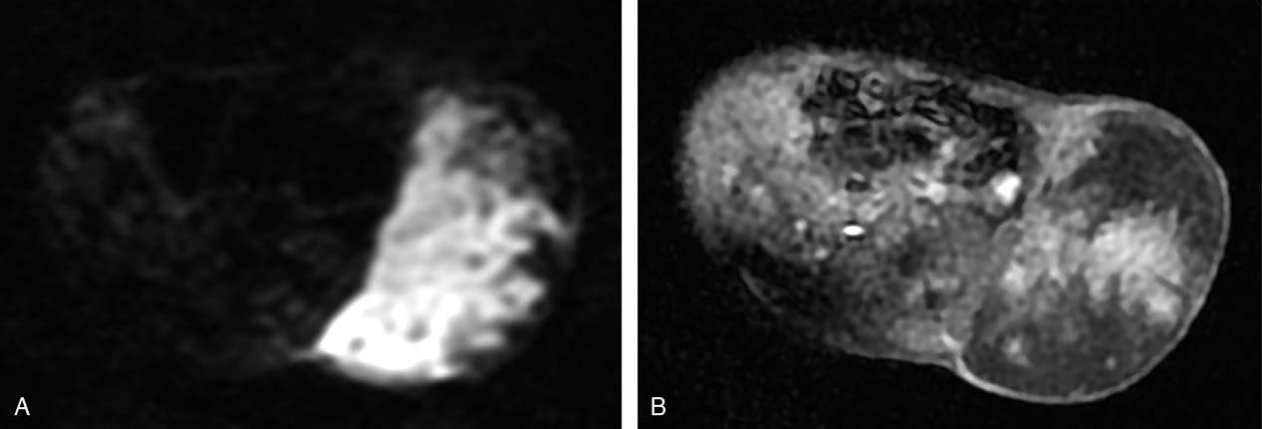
On postnatal axial DWI (b = 800 s/mm^2^), the mass showed marked hyperintensity similar to that observed in prenatal imaging (A). Enhanced axial T1-weighted imaging showed a heterogeneously enhanced mass (B).
